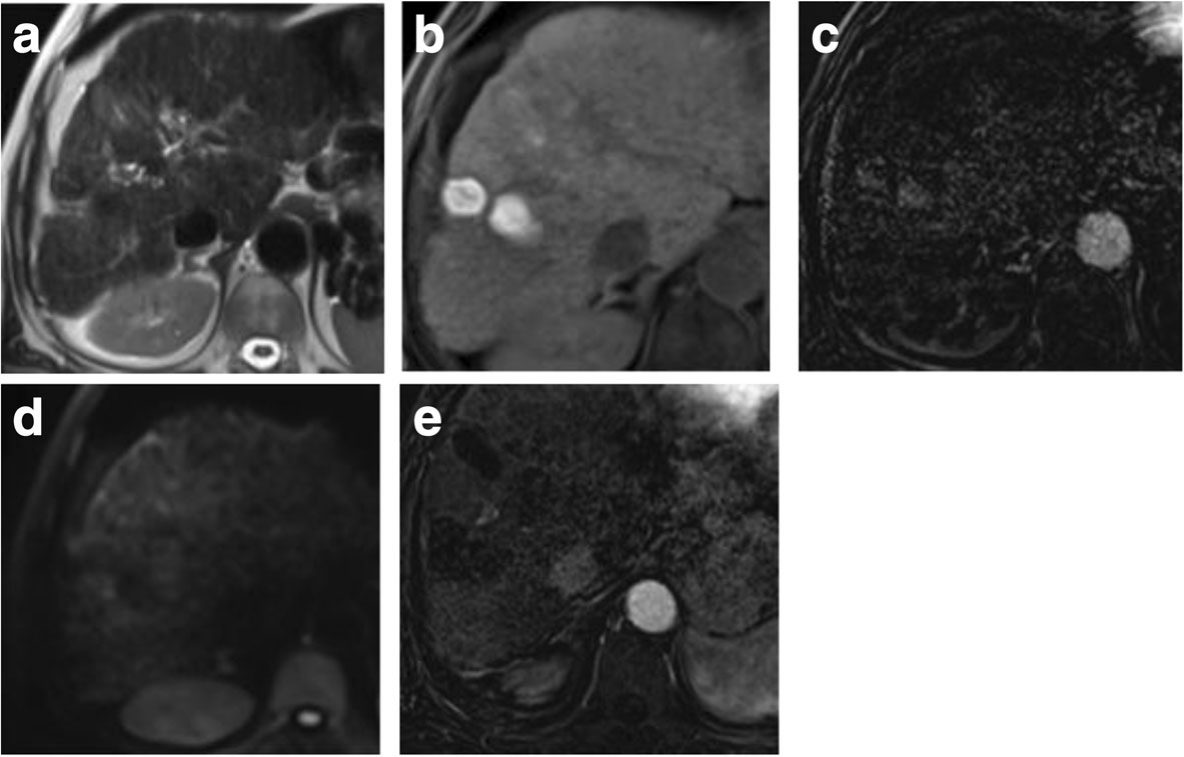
Two hepatic focal lesions after old RFA. a T2-WI shows a hypointense signal of the treated focal lesions. b Pre-contrast T1 fat-suppressed image shows the ablated HFLs with their bright signal due to coagulative necrosis. c Subtracted T1-WI arterial phase shows newly developed faint enhancement of the lesions. d DWI shows no true restriction corresponding to the enhancement. e Subtracted T1-WI arterial phase after a 3-month interval shows no enhancement of the lesions. Initial post-treatment assessment by LI-RADS v2018 is LR-TR equivocal that proved to be nonviable after a short-interval follow-up. DWI was consistent with LR-TR nonviable from the start with no restriction initially and on the follow-up (not shown)
